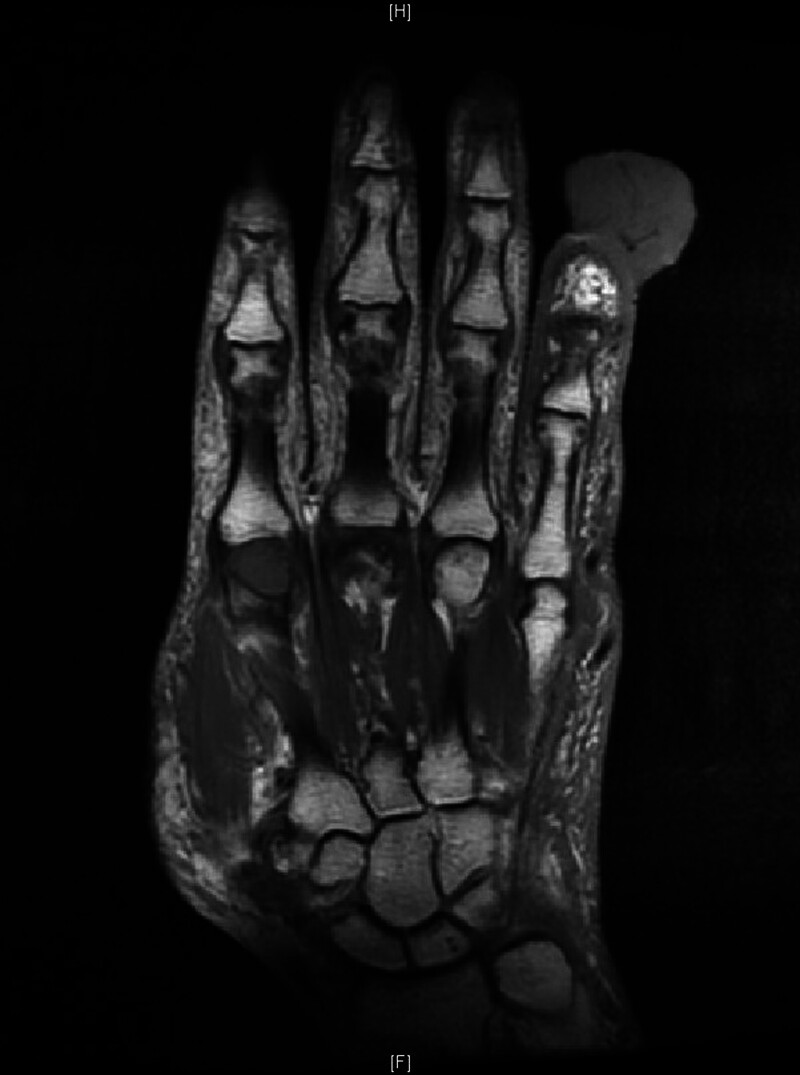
MRI of the left hand after finger resection showing resting metastatic lesions.
